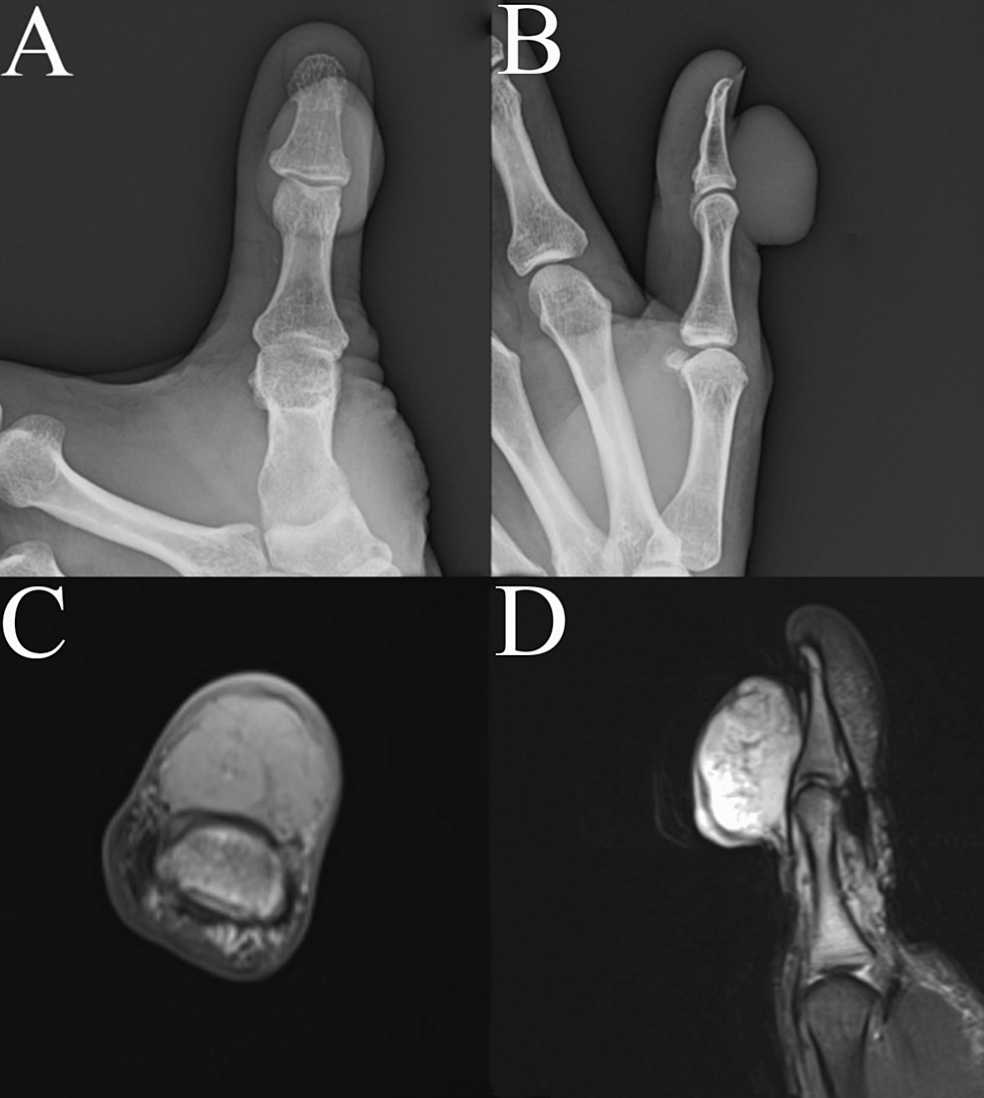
Imaging test results A: Posteroanterior; B: Lateral X-ray of the left thumb; C: Axial; D: Sagittal T2 fat suppression MRI of the left thumb showing an exophytic mass centered at the level of the interphalangeal joint.
